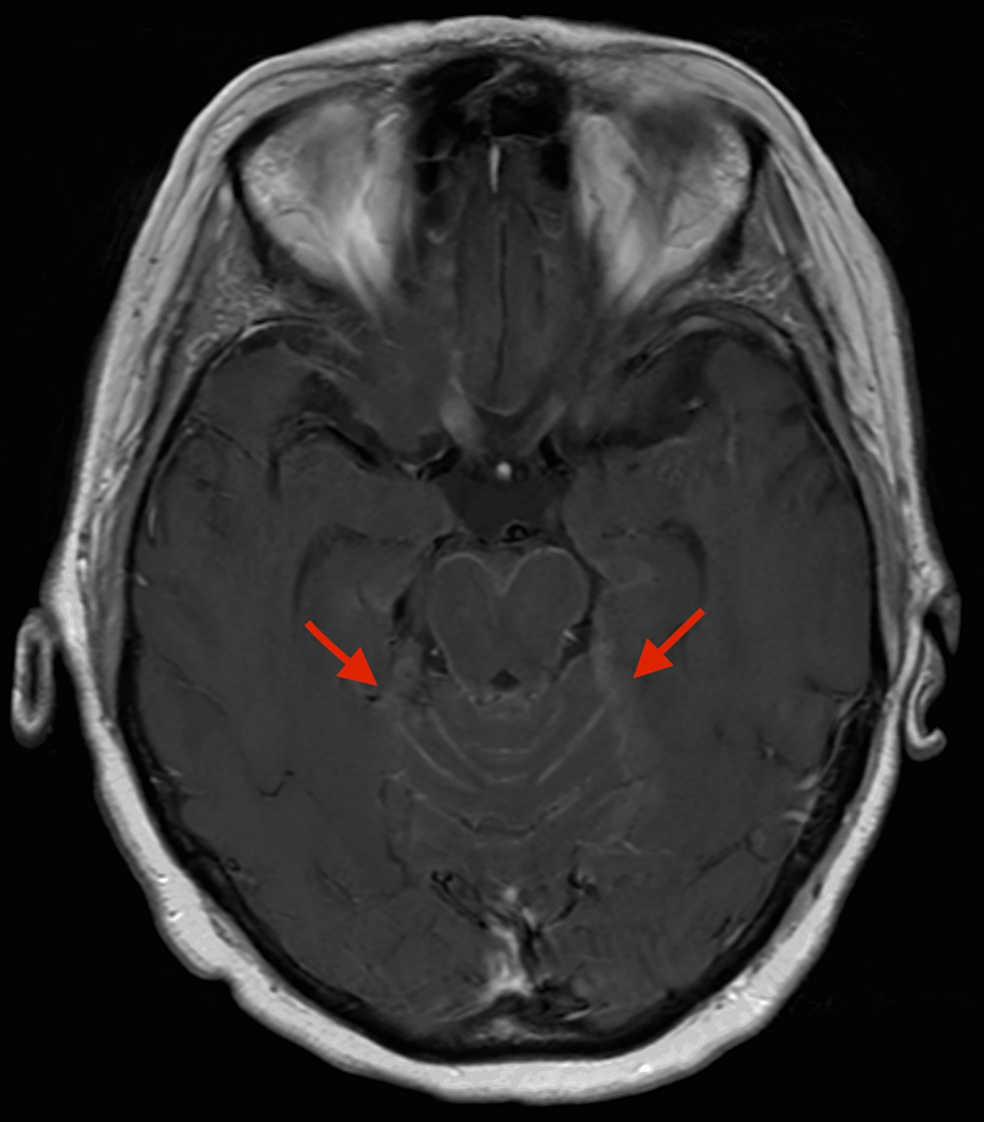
Brain MRI axial view during T1 post-contrast showing interval increase in leptomeningeal enhancement (red arrows) during admission. MRI: magnetic resonance imaging
